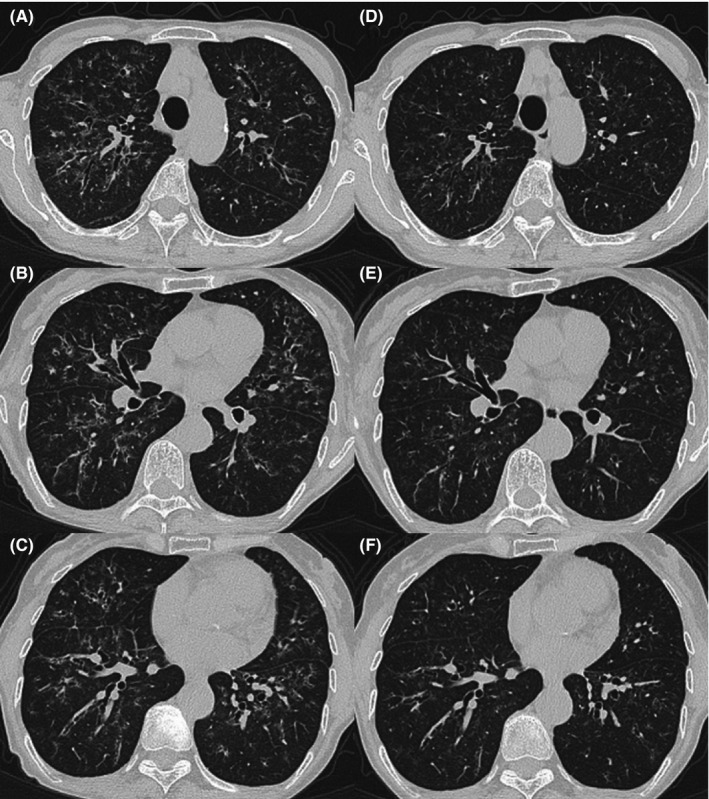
Chest CT on the day of admission showed ground‐glass opacities bilaterally along the bronchovascular bundles, bronchiectasis, and bronchial wall thickening (A–C). After treatment, these findings improved (D–F)
